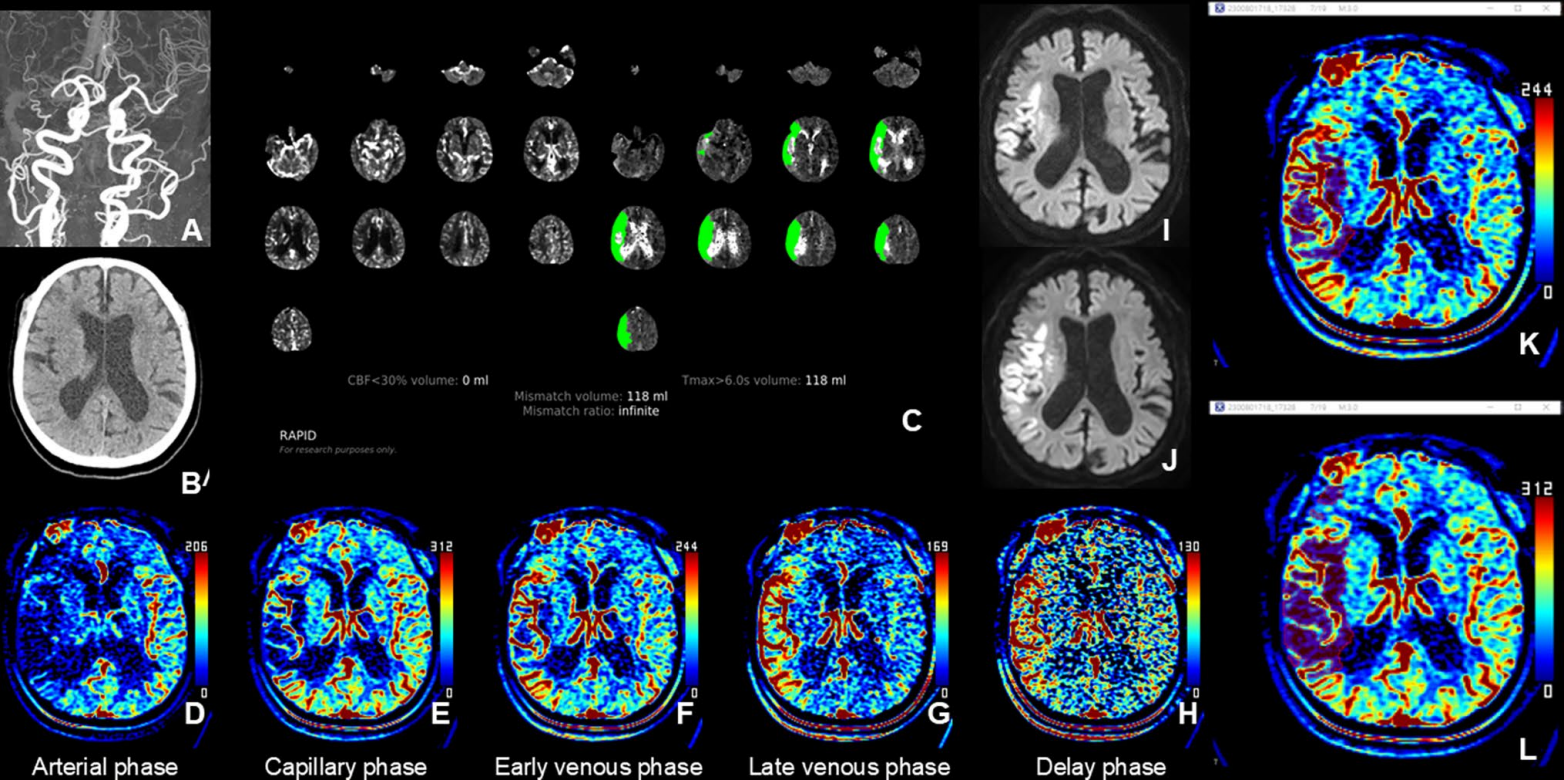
Images of an 82-year-old woman with occlusion of the right middle cerebral artery (MCA) demonstrated on CT angiography (A). This patient had a premorbid modified Rankin Scale (mRS) score of 0, and her National Institutes of Health Stroke Scale (NIHSS) score at admission was 12. She received intravenous thrombolysis followed by intraarterial thrombectomy, resulting in complete recanalization of the occluded artery. Her 90-day mRS score was 3. Upon admission, a brain CT scan (B) showed indistinct differentiation between the cortical gray matter and white matter in the right temporal and insular regions, making it difficult to precisely determine the extent of the baseline lesion. CT perfusion analysis using RAPID software (RAPID, RapidAI®, Menlo Park, CA, USA) (C) indicated a baseline lesion volume of 0 ml, as measured by a relative cerebral blood flow threshold of < 30%, meaning the baseline lesion could not be identified. The CT collateral map, generated from the CT perfusion images (D–H) at admission, indicated poor collateral perfusion (collateral perfusion score of 1: collateral perfusion delay involving more than half of the MCA territory in the early venous phase and less than half in the late venous phase). Diffusion-weighted imaging (DWI) (I), performed immediately after the CT scan, revealed acute infarct signals in the right MCA territory. The extent of the DWI lesion at admission closely corresponded with the hypoperfused area visible up to the early venous phase on the CT collateral map (F). This suggests that even without DWI, the baseline lesion can be identified using a CT collateral map. The baseline lesion shows slight evolution on DWI on day 1 after recanalization of the occluded MCA (J). K and L are images shown on Medical Image Processing, Analysis, and Visualization (MIPAV; version 7.1.1; National Institutes of Health). These images display the hypoperfused lesion highlighted in the early venous (K) and capillary (L) phases, respectively, for assessing the hypoperfused lesion volume on the collateral map
